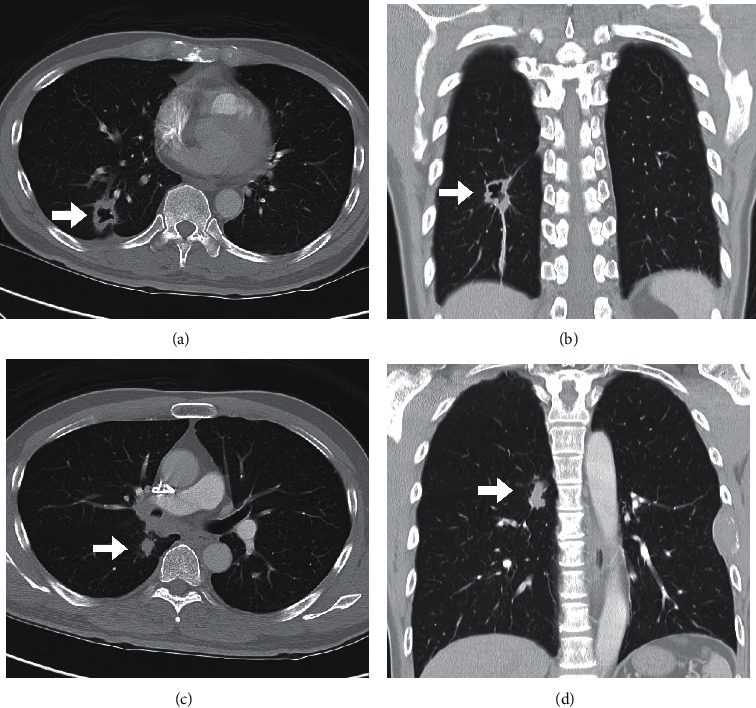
Low-dose lung CT demonstrated multiple right lung tumors (arrow).
